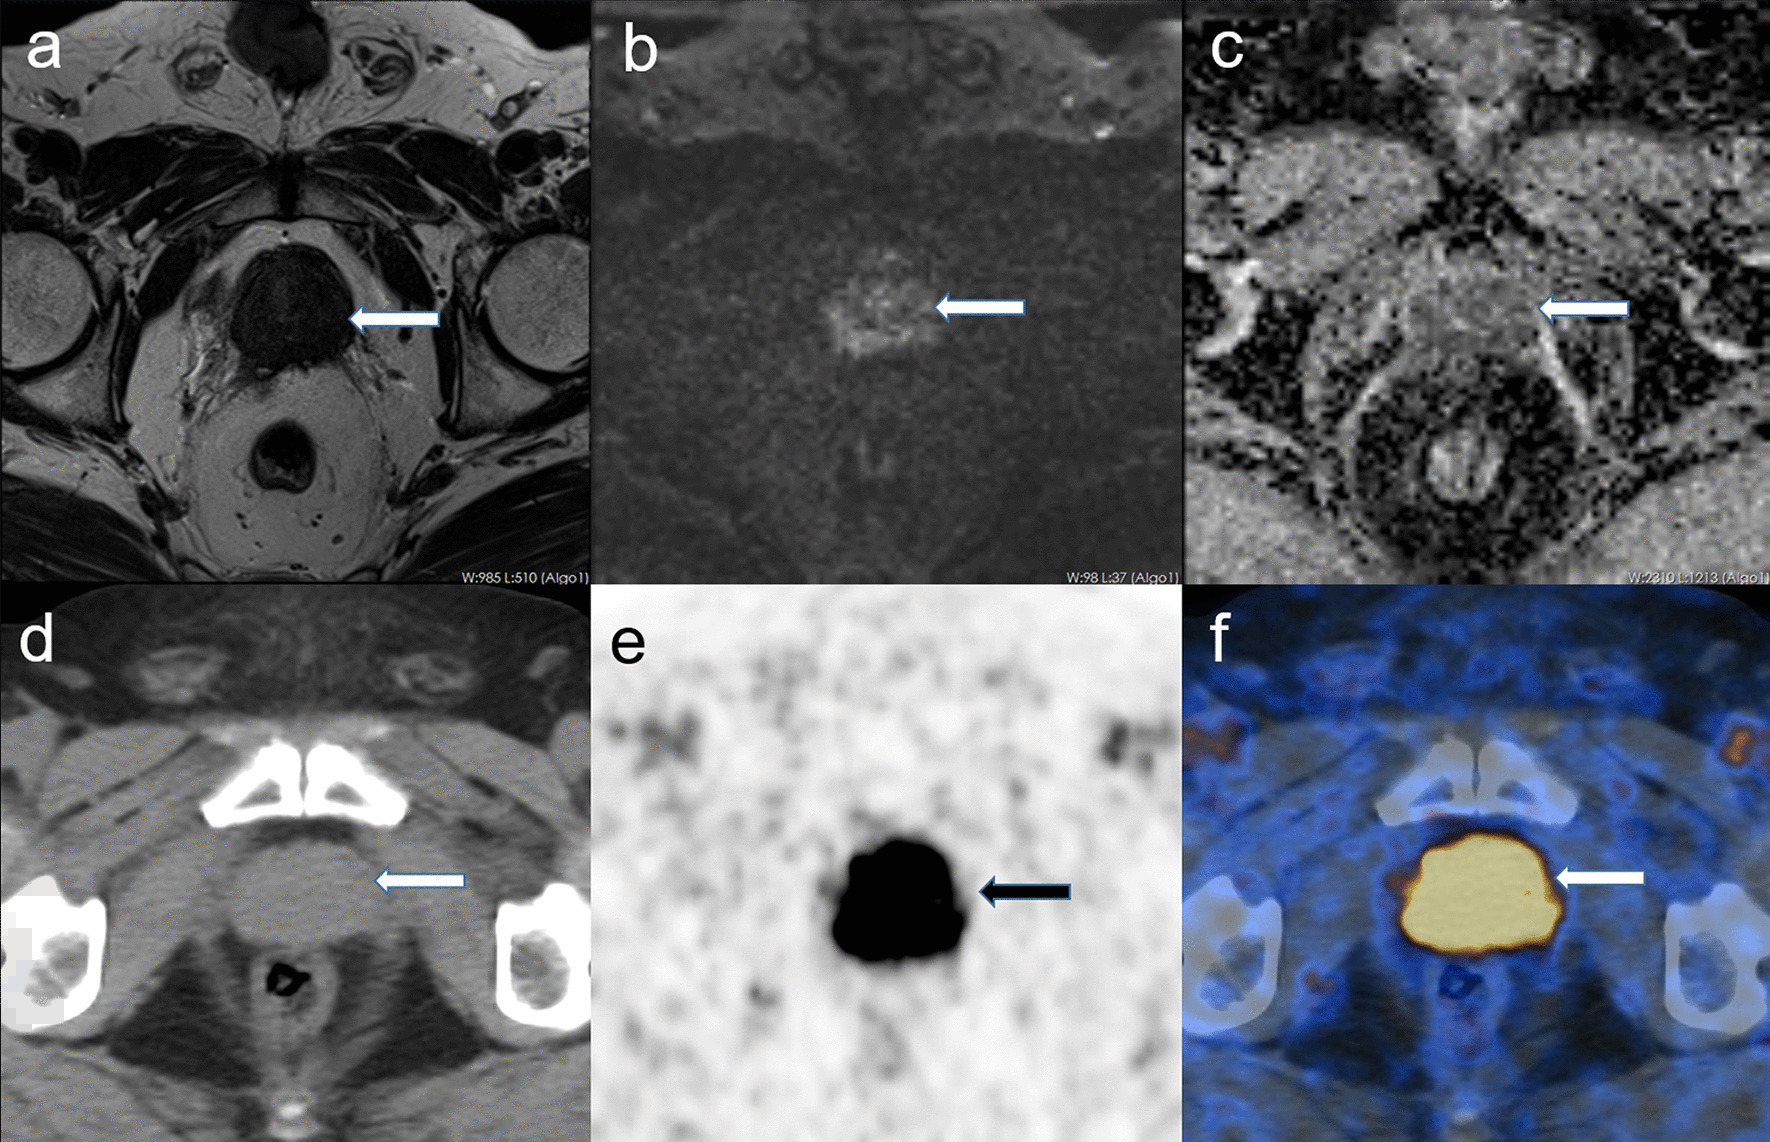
High-risk PCa with mpMRI ( −) and ^68^Ga-PSMA PET/CT (+). A 69-year-old patient whose serum PSA level was 15.4 ng/ml and whose GS was 5 + 5. There is no significant lesion in the prostate gland in the pelvic MRI scan (a T2w; b b 1500 DWI), while in the ^68^Ga-PSMA PET/CT there is a strong tracer uptake highly likely to be diagnosed (arrow in e and f). a–c MRI; d–f
